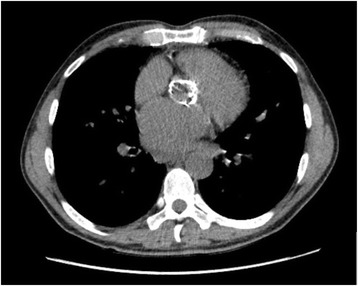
Preoperative computed tomography scan displaying a severe circumferentially calcified aortic annulus
